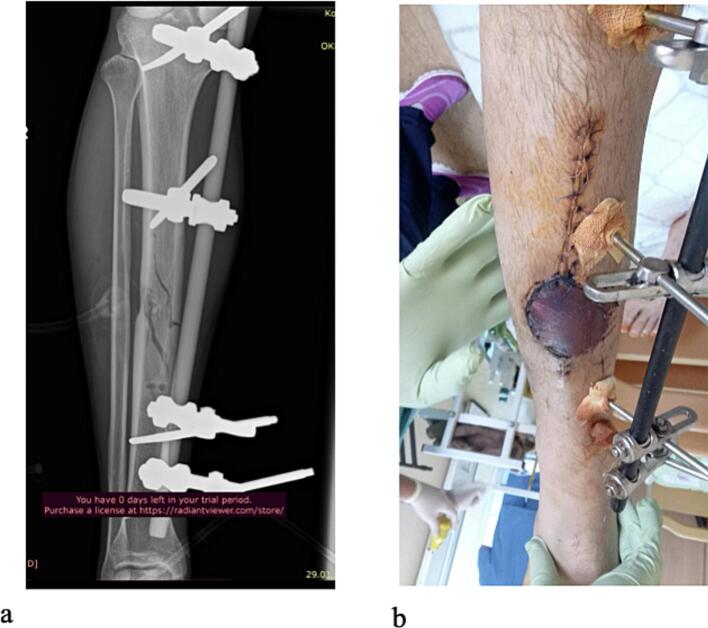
Clinical information from the previous hospital. a. Plain radiographs. b. Postoperative image after the flap surgery.
